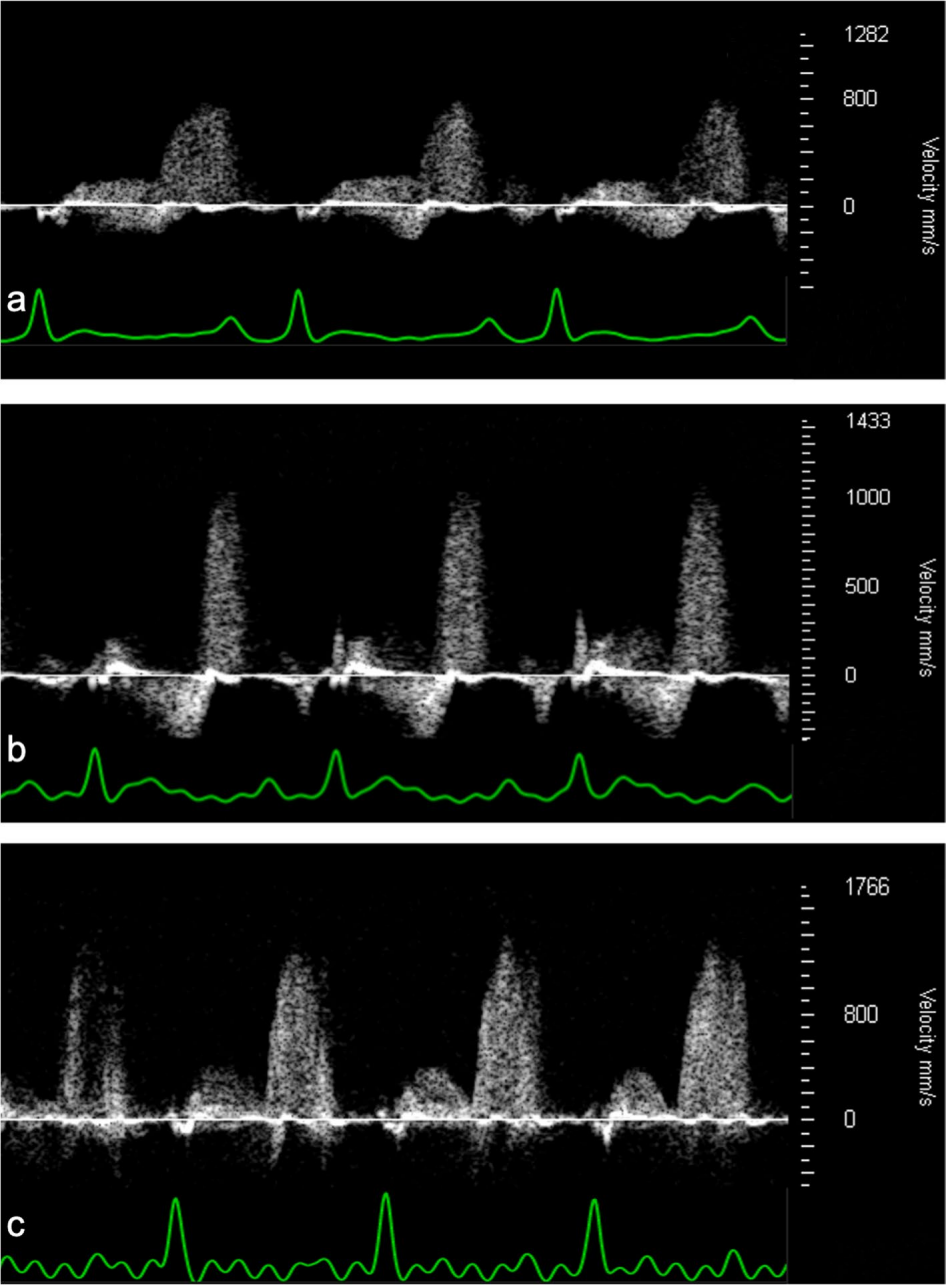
Representative pulsed-wave Doppler coronary flow patterns. The signals are shown with the corresponding electrodardiographic signal during baseline conditions (a), infusion of 140 μg/kg body weight/min adenosine (b), and infusion of 10 μg/kg body weight/min dobutamine (c)
